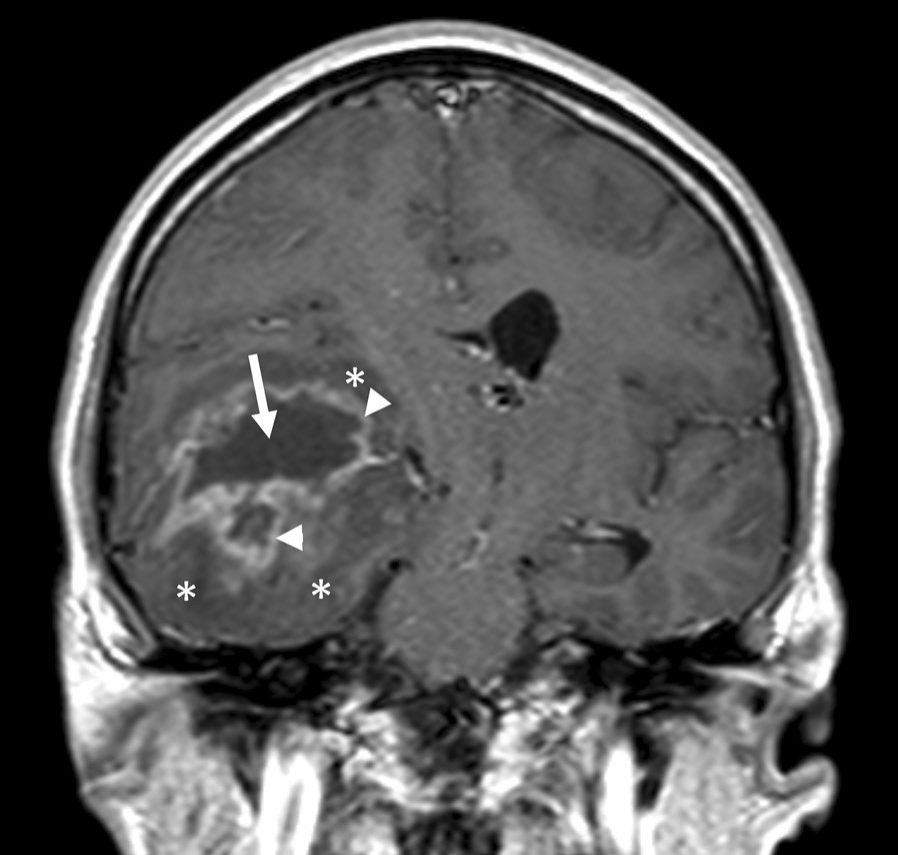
Magnetic resonance image (MRI) of cystic glioblastoma. A T1-weighted MRI obtained after intravenous infusion of a gadolinium-based contrast agent shows a contrast-enhancing cystic glioblastoma in the right temporal lobe. The arrow indicates the cyst. Arrowheads indicate contrast-enhancing tumor, in which leaky capillaries allow the contrast agent to escape into the tumor tissue. Asterisks indicate the peri-tumoral edema, which appears darker than the surrounding brain tissue
